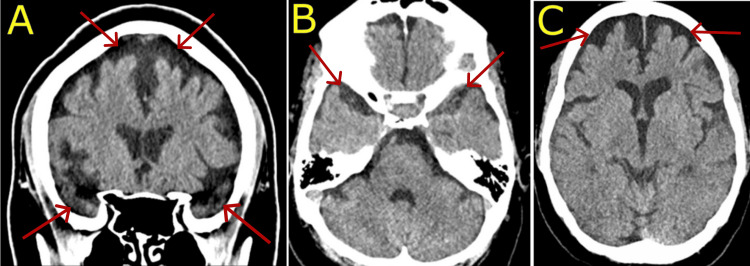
A) Coronal CT image showing diffuse cortical atrophy (red arrows, above) and loss of temporal lobe density (red arrows, below). B) Axial CT image showing loss of temporal lobe density (red arrows). C) Axial CT image showing diffuse cortical atrophy (red arrows).
